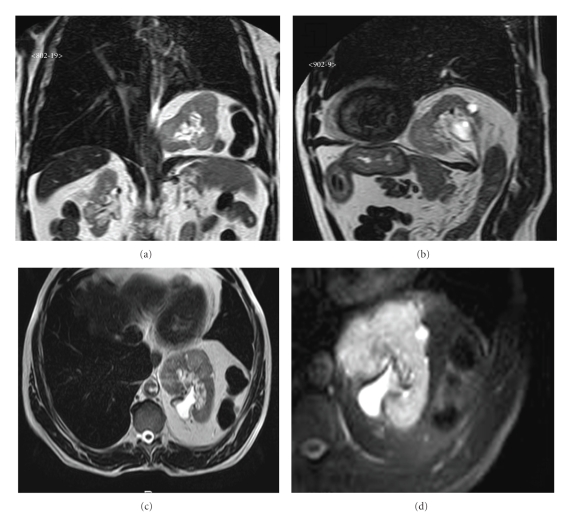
Magnetic resonance imaging (MRI). (a) T2 sequence on coronal view. (b) T2 sequence on sagittal plane. (c) T2-weighted image on axial plane. (d) Particular of left kidney on T2 axial view with fat suppression.
